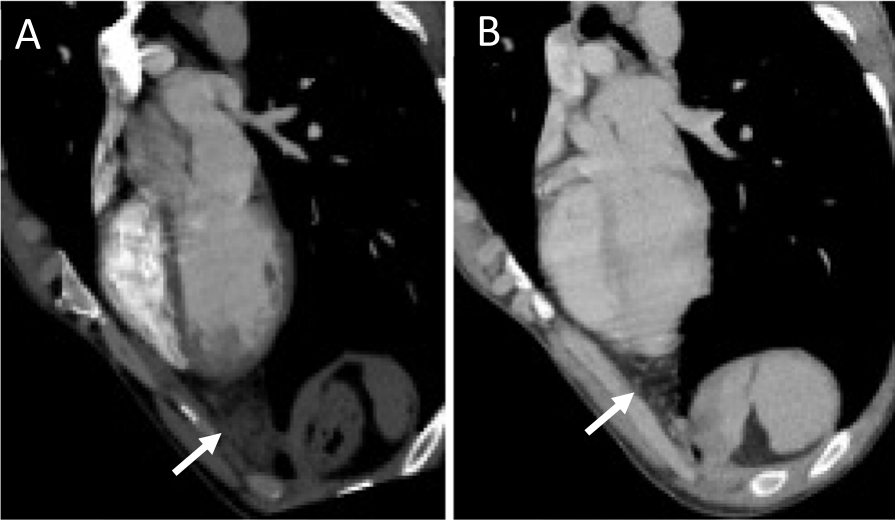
Comparison of contrast enhanced chest CT at the time of clinical presentation (A) and after two months (B). Reformatted axial oblique images demonstrating resolution of the epicardial fat stranding of the left cardiophrenic angle (white arrows)
